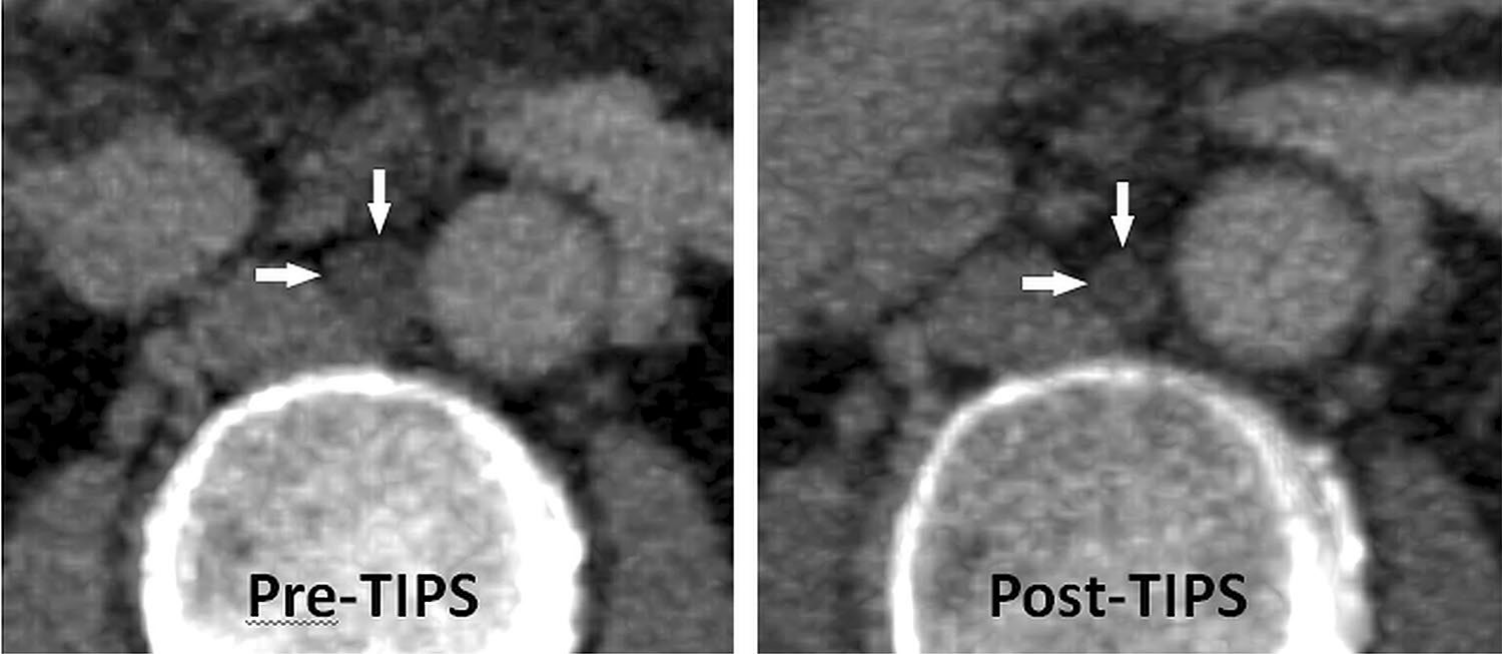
CT images of a patient before and after TIPS-creation demonstrating a considerable decrease in cisterna chyli (arrows) diameter after TIPS-creation.
